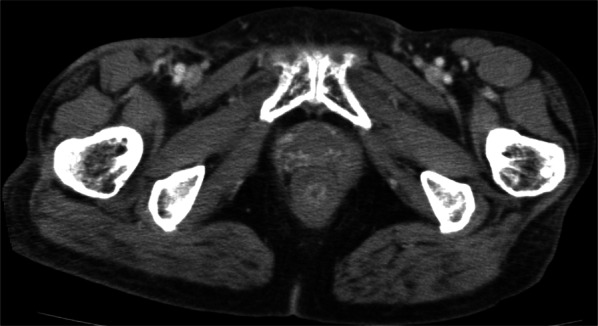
Axial computed tomography slice after chemoradiotherapy. Primary lesion and pelvic and inguinal lymph nodes achieved complete response
